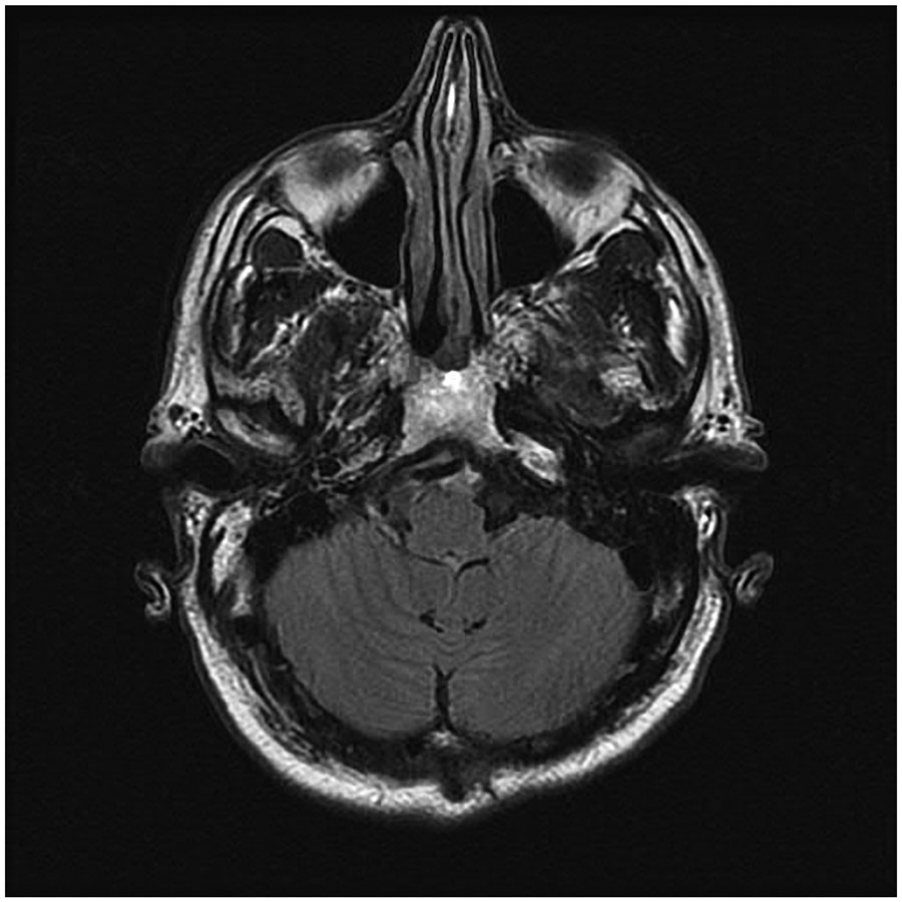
Post-operative (4 months) T2 flair axial magnetic resonance image (MRI) showing absence of lesion.
